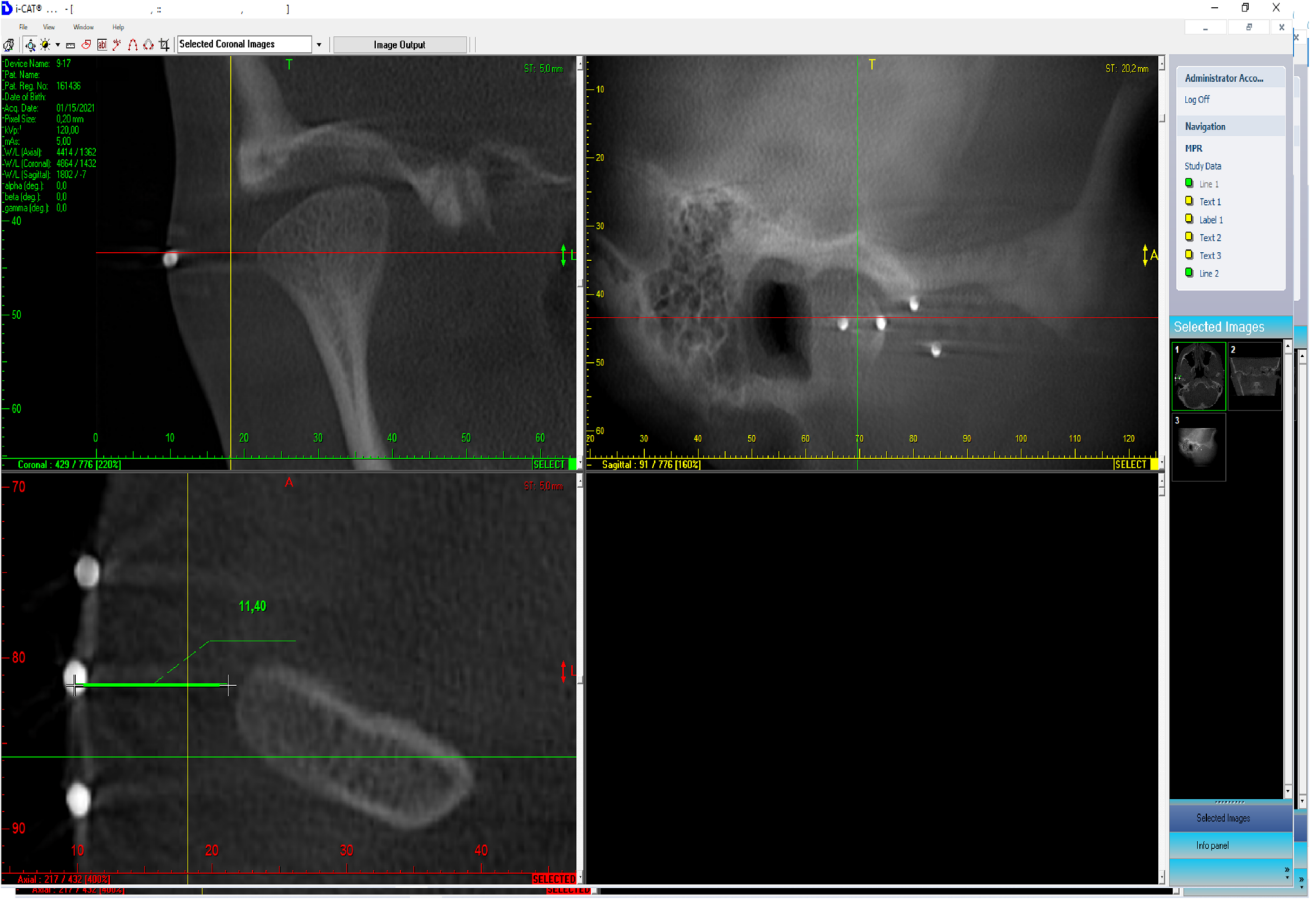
CBCT image*s* showing the correspondence *between* the marked points and *the* osseous joint components.
